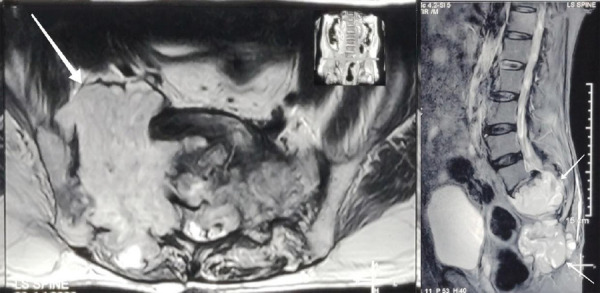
Axial section of MRI of the LS spine with a huge expansile mass in the upper sacrum (white arrow) (A), Sagittal section of T2 weighted MRI of the LS spine showing the mass (white arrows) (B).
